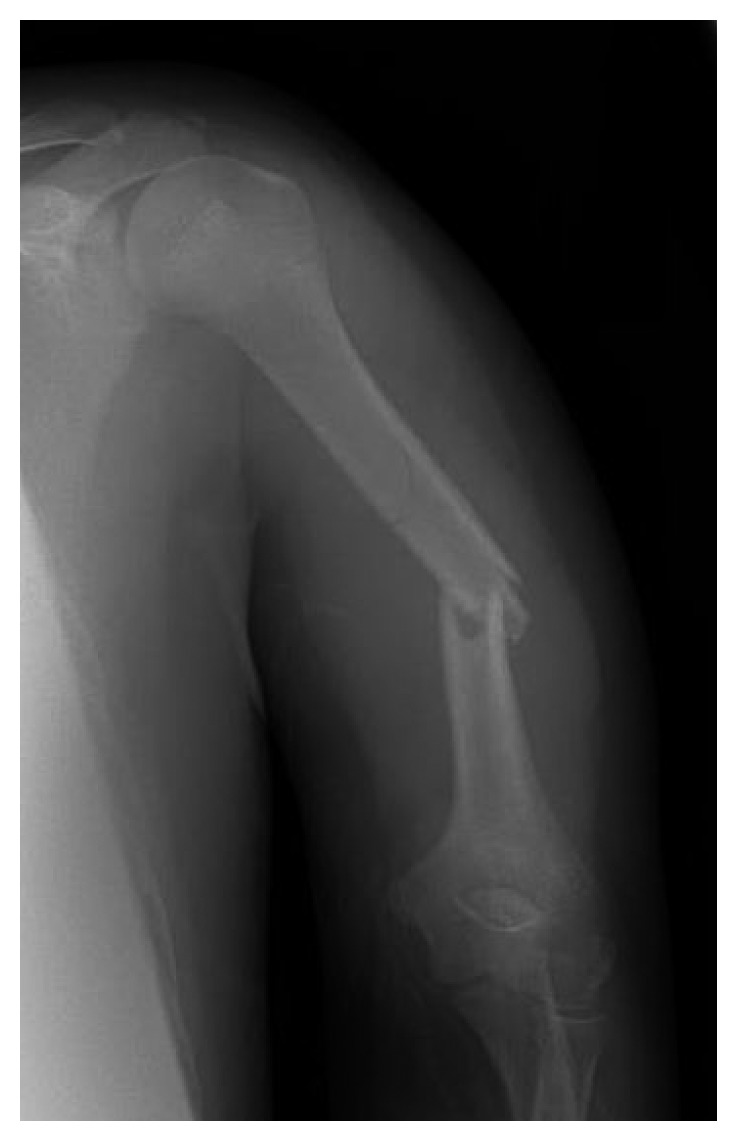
Anteroposterior radiograph showing a displaced midshaft humerus fracture in 35° varus angulation.
